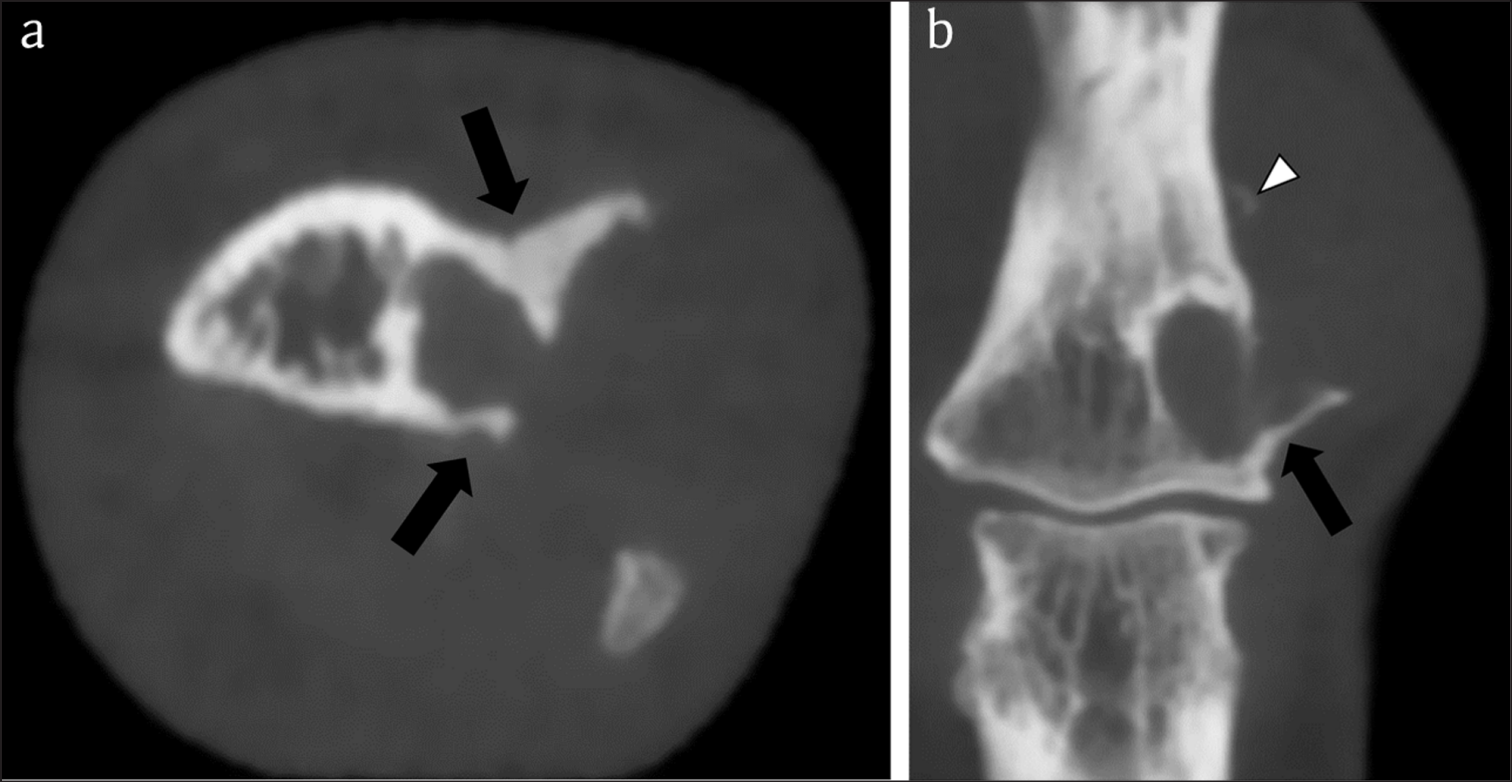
Case 2 – CBCT. Axial image (a). Coronal reformatted image (b). The lesion consists of a smaller intramedullary and a larger juxta-cortical component. The intramedullary part is well-delineated by a rim of peripheral sclerosis, whereas the juxta-cortical part causes scalloping of the outer cortex within overhanging edges (black arrows in a and b). The cortical breakthrough and overhanging margins are far better demonstrated than on CR (see Figure 6). Note a small fleck of calcification (white arrowhead), supporting the hypothesis that the lesion contains chondroid matrix.
